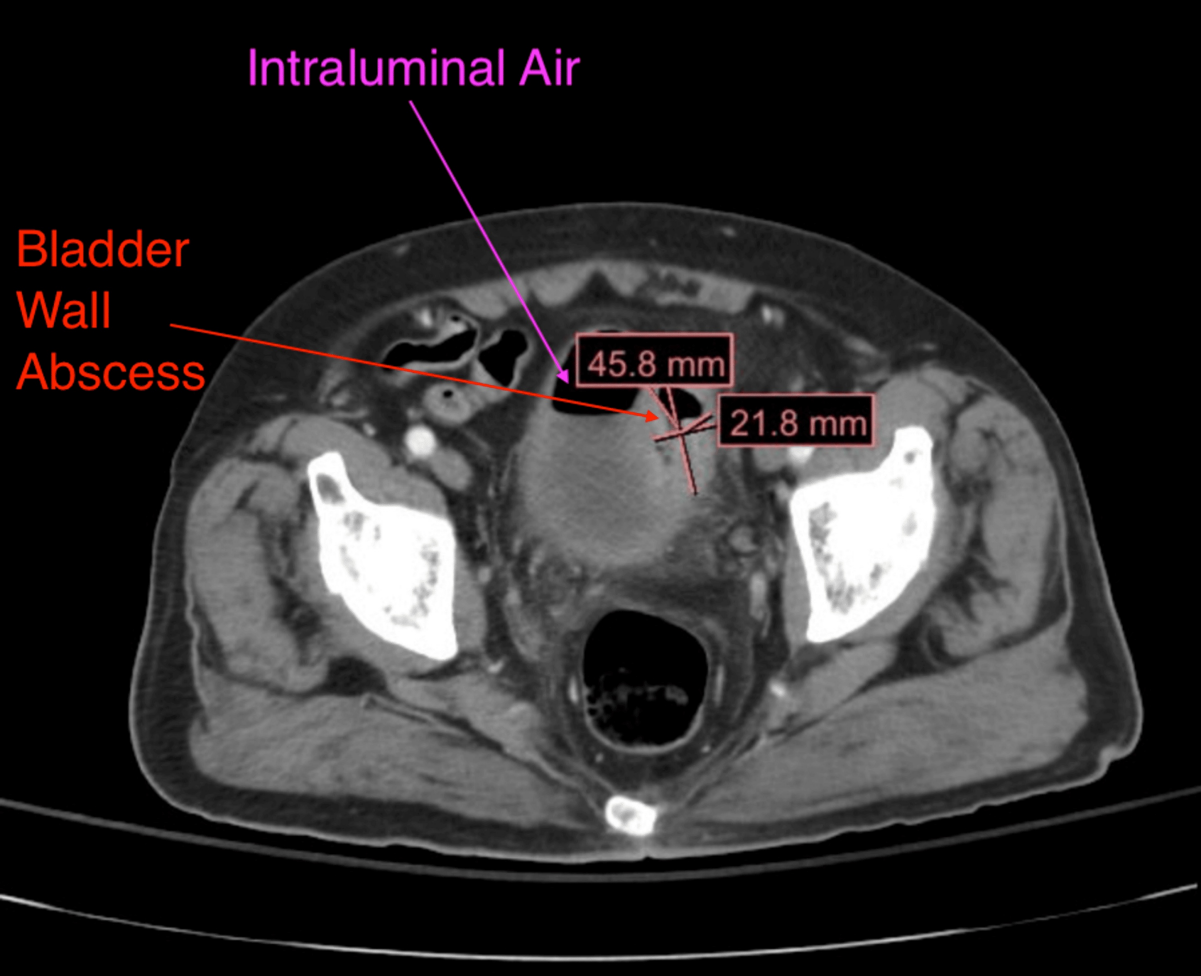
CT (axial view) showing a large bladder wall abscess and intraluminal air communicating from the large intestine
